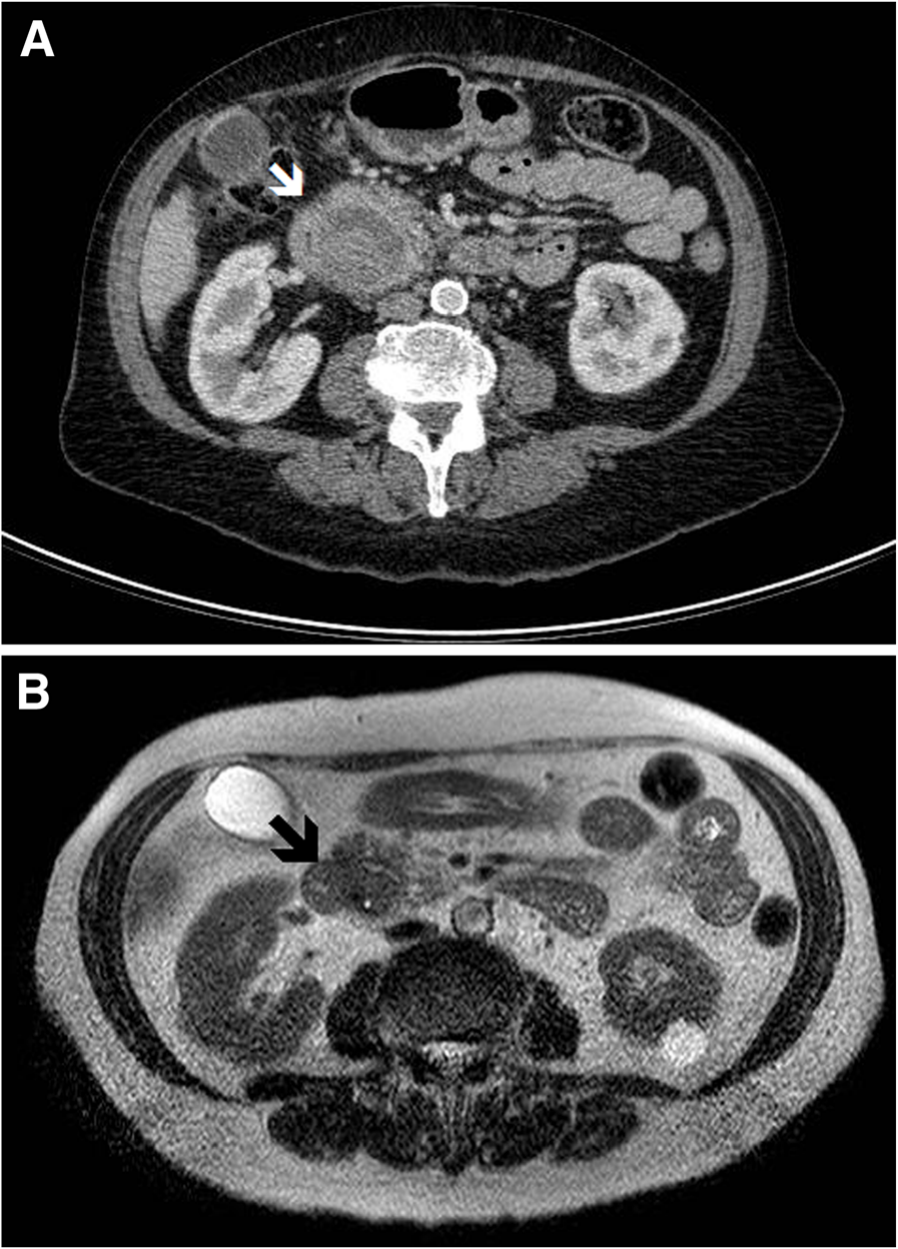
CT and MRI images of the second patient. (A) A large heterogenous mass (white arrow) was identified at the head of pancreas. This was identified as a haematoma. (B) Follow-up MRI showed the pancreatic mass (black arrow) had decreased in size and demonstrated characteristic signals for a haematoma.
